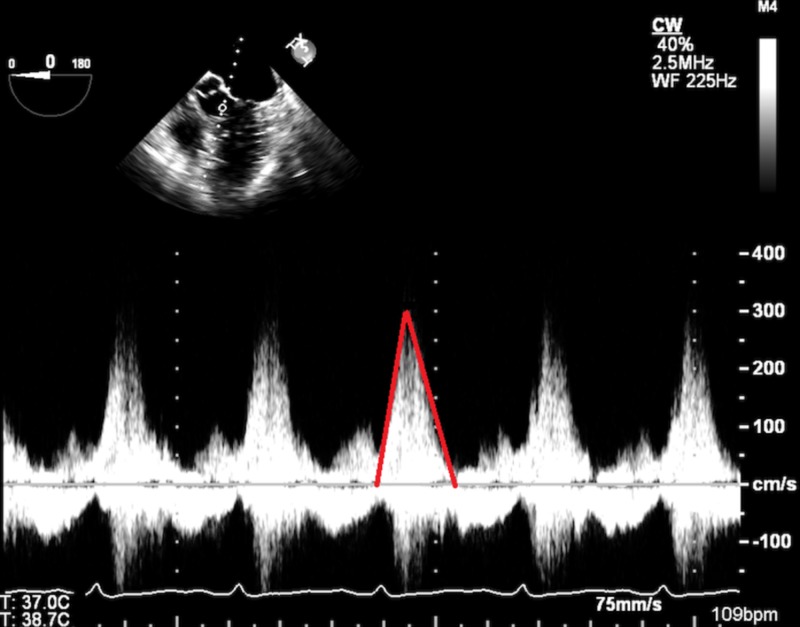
Transesophageal echocardiographic image showing increased left ventricular outflow tract velocity with continuous wave Doppler (red outline)
